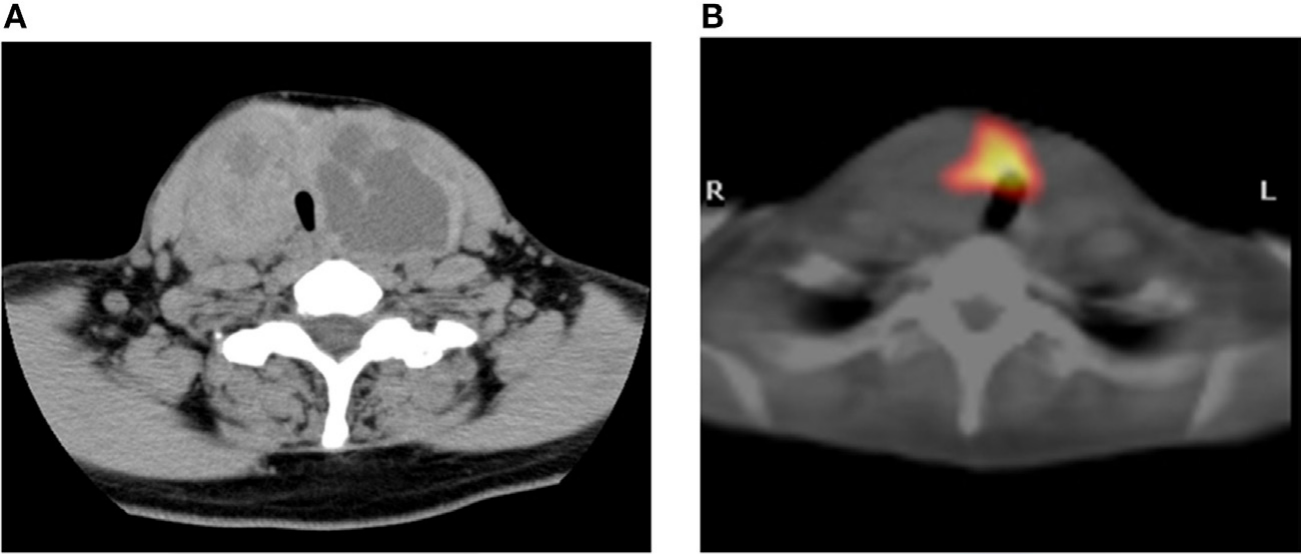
Imaging study. CT scan showing multinodular enlargement of the thyroid gland (A). The corresponding region of radioiodine uptake represents cold nodules in this SPECT/CT fusion image (B).
